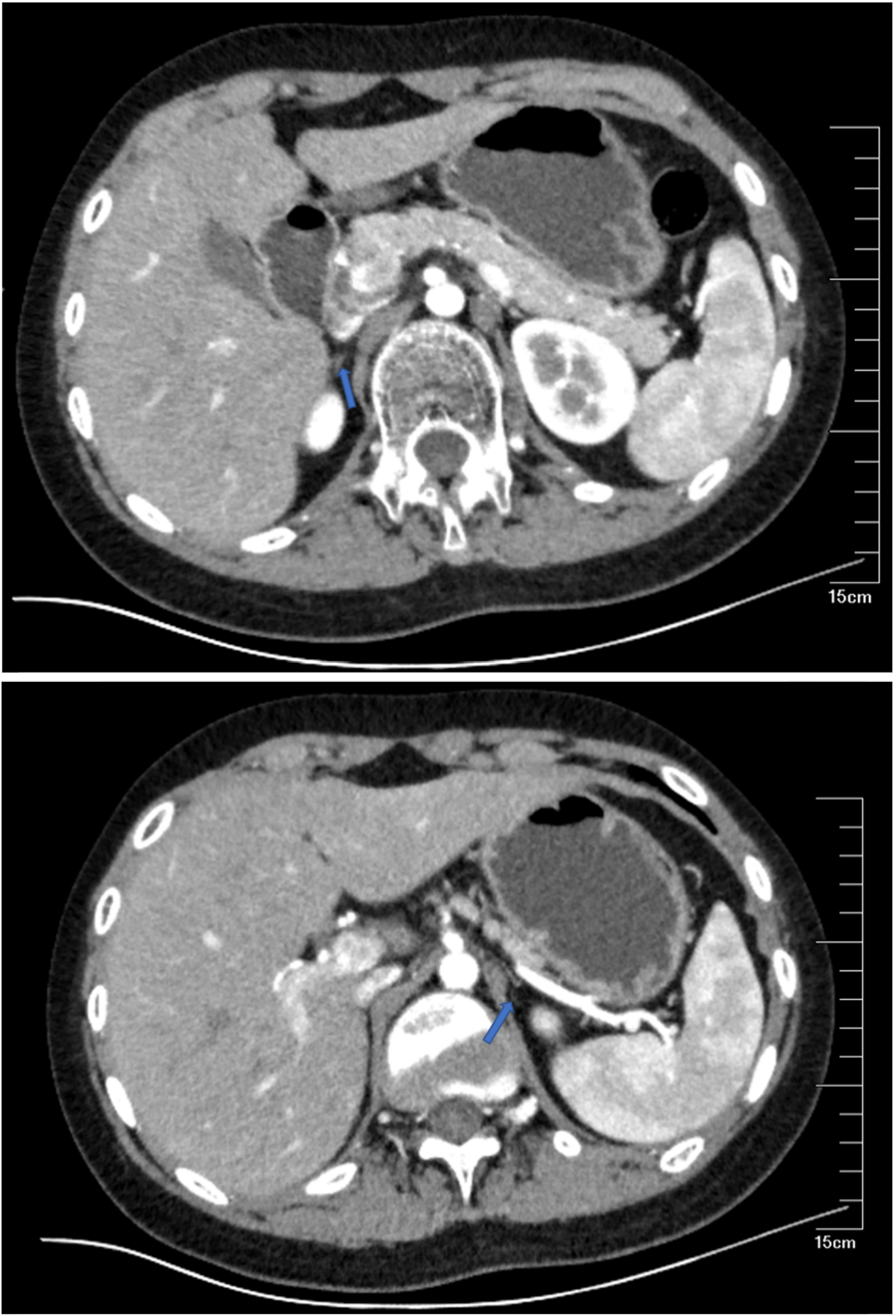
The results of bilateral adrenal enhanced CT
